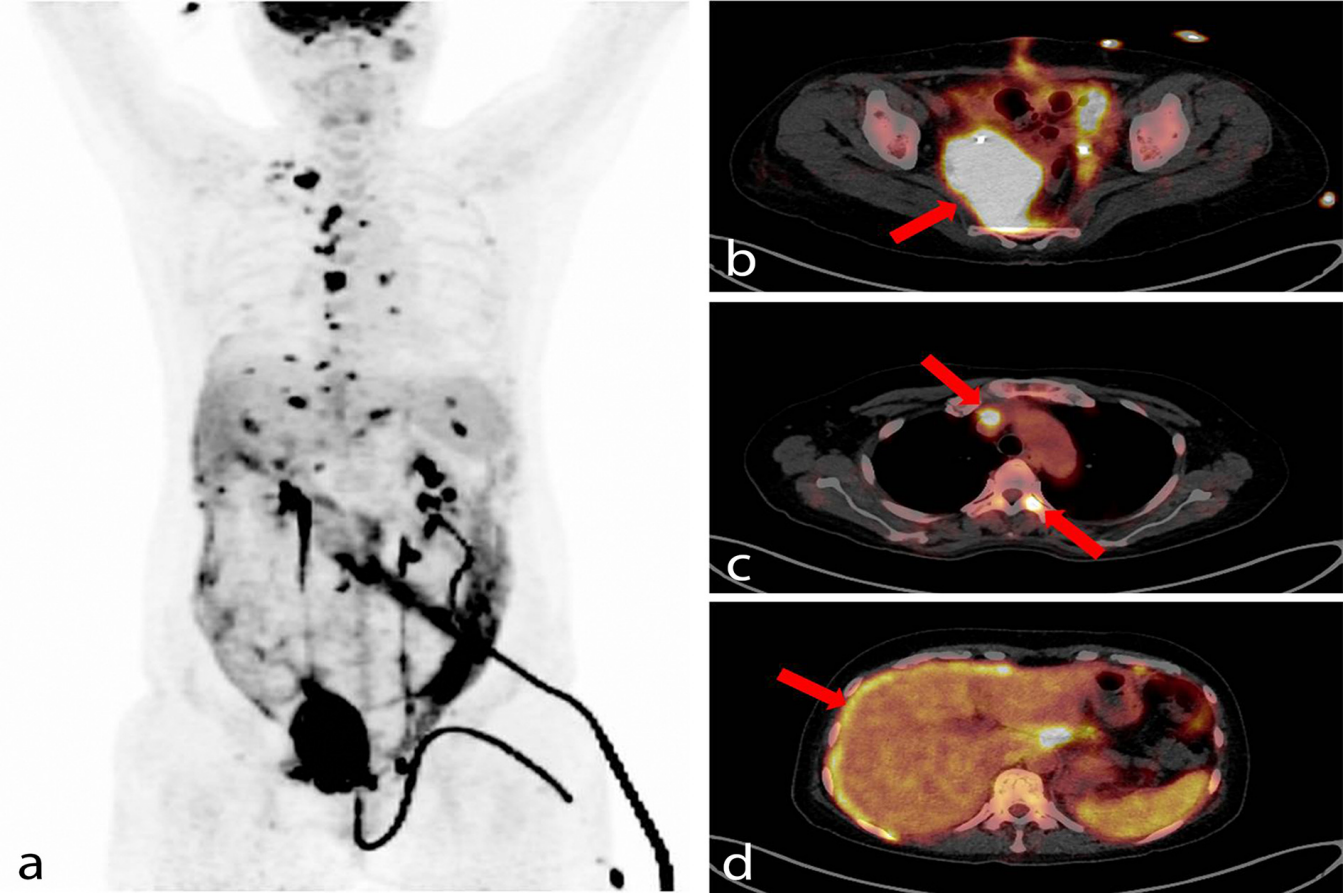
(a, b arrow) A ^18^F-fludeoxyglucose positron emission tomography/CT scan (Biograph mCT 128, Siemens, Germany) was performed for evaluation of the huge pelvic mass. The maximum intensity projection and fusion axial images show a huge intense hypermetabolic mass arising from the uterus with a maximum standardized uptake value of 37.1 and extending to the right pelvic side wall, which suggests a malignancy of uterus. (c, d arrows) Other multifocal or diffuse hypermetabolic lesions were observed in the intraperitoneal space, bones, neck and thoracic lymph nodes, suggesting multiple malignant involvement with peritoneal seeding.
